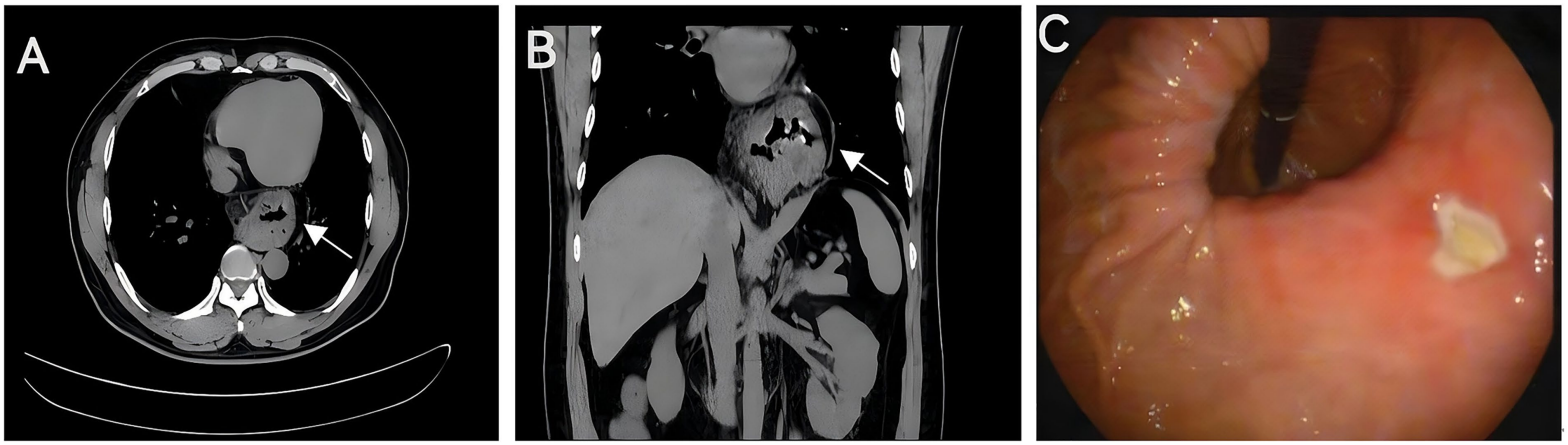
(A) Plain CT imaging demonstrates partial gastric herniation into the thoracic cavity, with the stomach positioned between the heart and abdominal aorta. (B) Coronal CT reconstruction more clearly delineates the herniation of gastric tissue into the thoracic cavity. (C) A large hiatal hernia is present, with a well-defined 5 mm × 6 mm ulcer observed along the lesser curvature of the upper gastric body, adjacent to the cardia.
